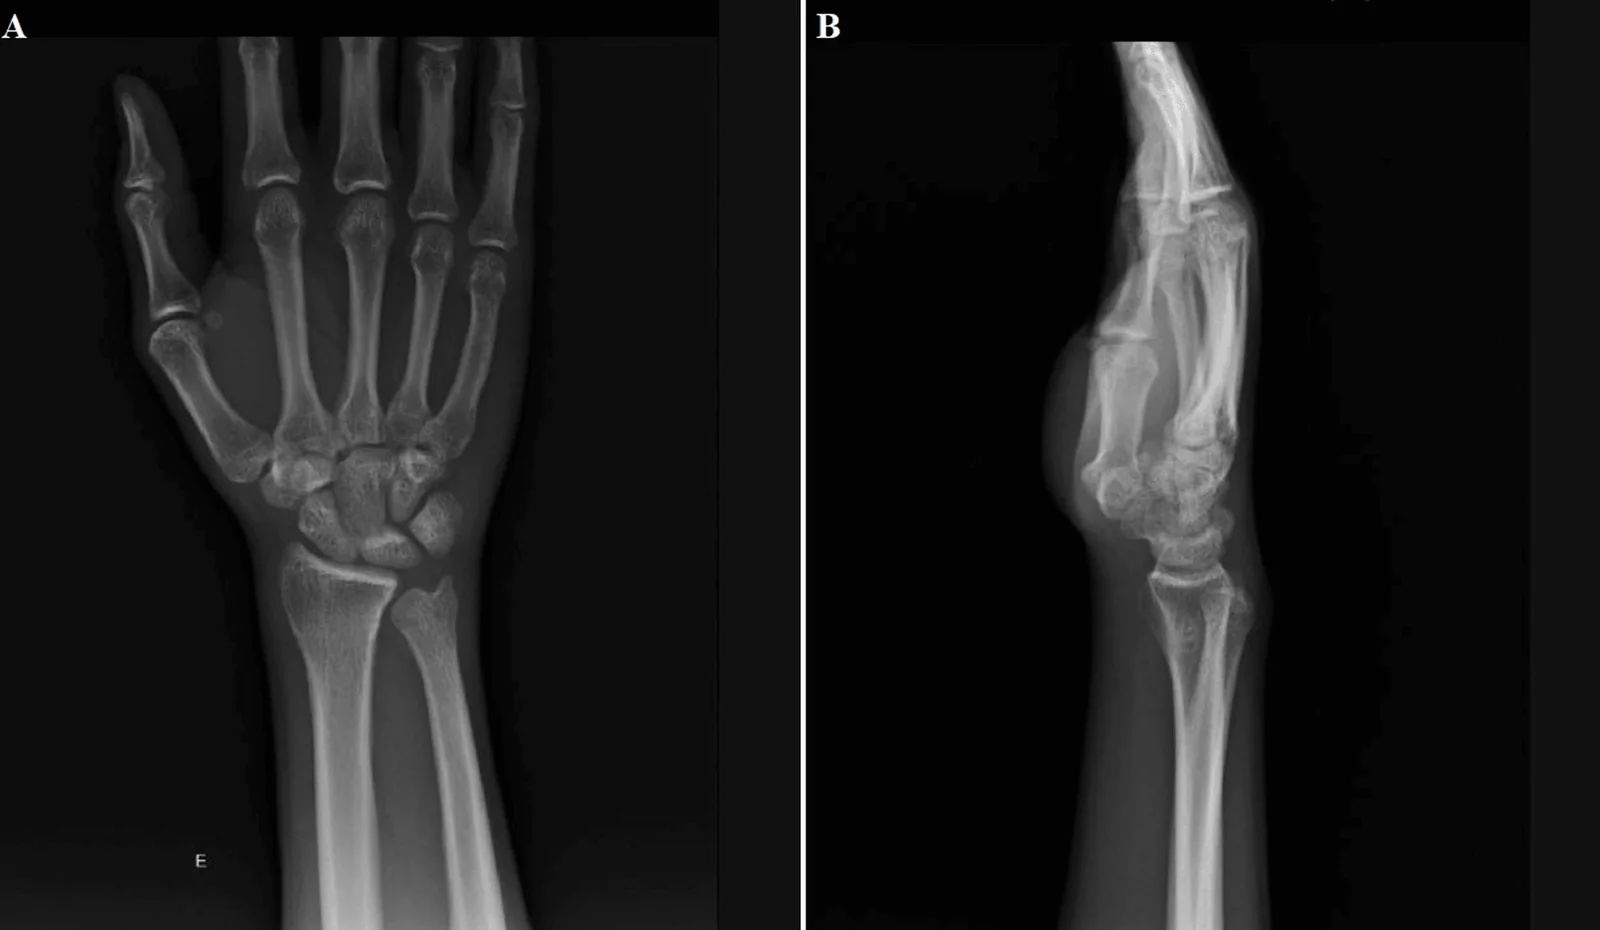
Radiographs at two-month follow-up. (A) Anteroposterior view. (B) Lateral view showing maintained DRUJ alignment after removal of fixation. DRUJ: distal radioulnar joint
